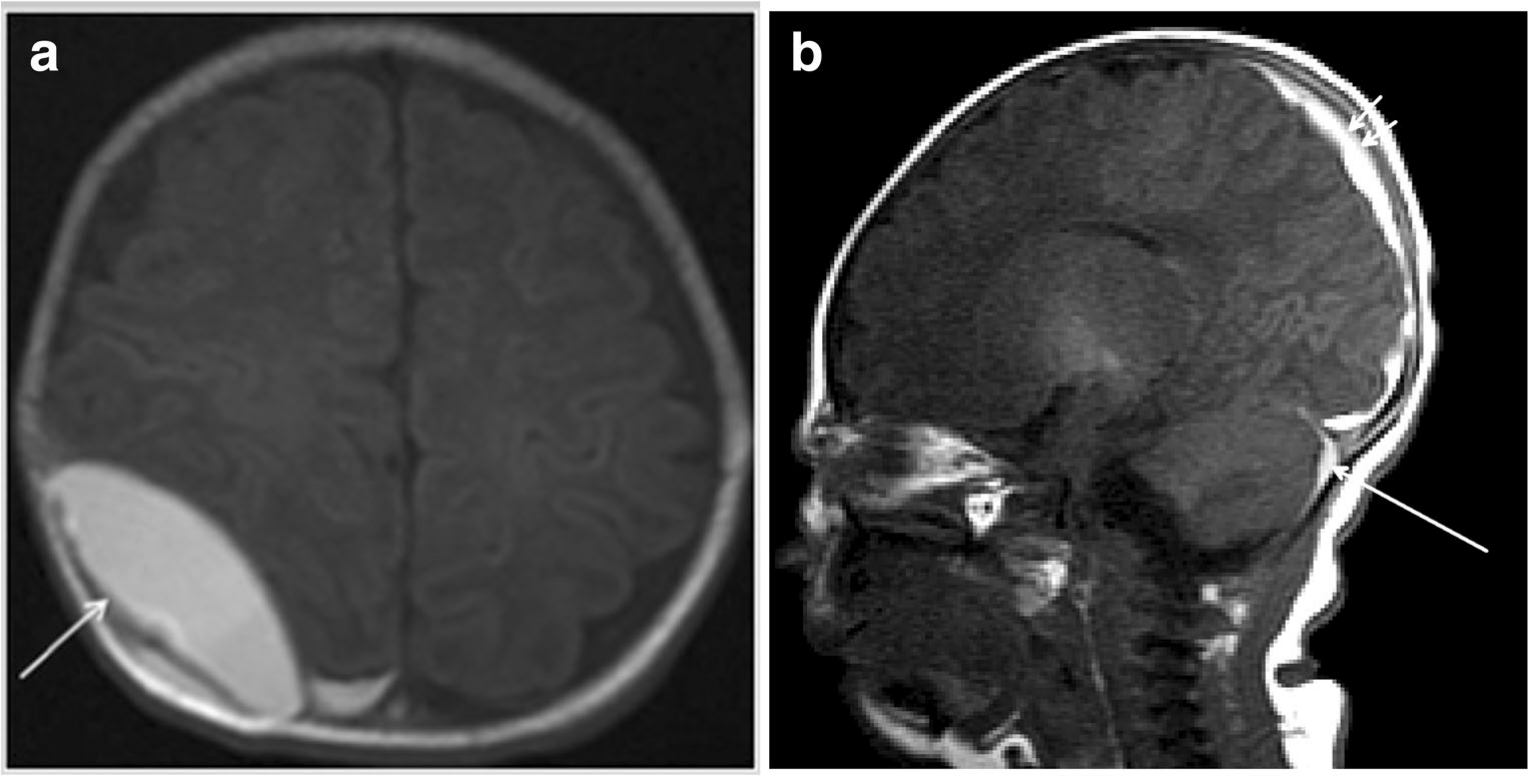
A 9-day-old female neonate exhibited both supratentorial and infratentorial SDH and EDH at the right parietal convexity. She was born via spontaneous vaginal delivery at 38 weeks weighing 3070 g. She presented with jaundice 4 days postnatally and exhibited a EDH (arrow) at the right parietal convexity on axial T1WI MR imaging and b SDH over both cerebellar and parietooccipital lobes (short arrows). She underwent burr hole drainage of EDH 11 days postnatally. She was followed in the clinic for 4 years and developed normally
